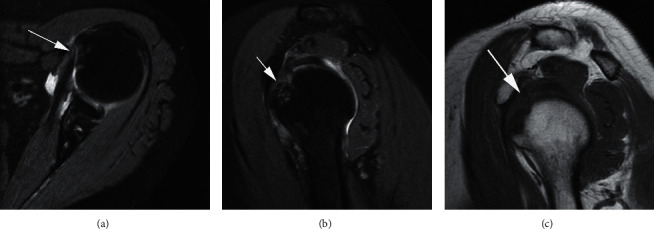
A 64-year-old woman with left shoulder pain after a fall. Axial (a) and sagittal oblique (b) T2-weighted FS MRI demonstrates a partial insertional tear of the subscapularis tendon (arrow). Sagittal oblique T1-weighted MRI (c) demonstrates loss of fat within the rotator interval (arrow).
